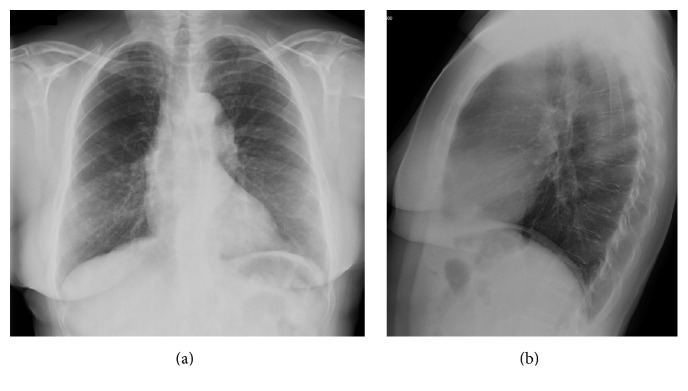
Posteroanterior (a) and lateral (b) chest X-rays. In a routine chest radiography 2 years before presentation in the ER it was difficult to detect the lesion in the left lower lung.
